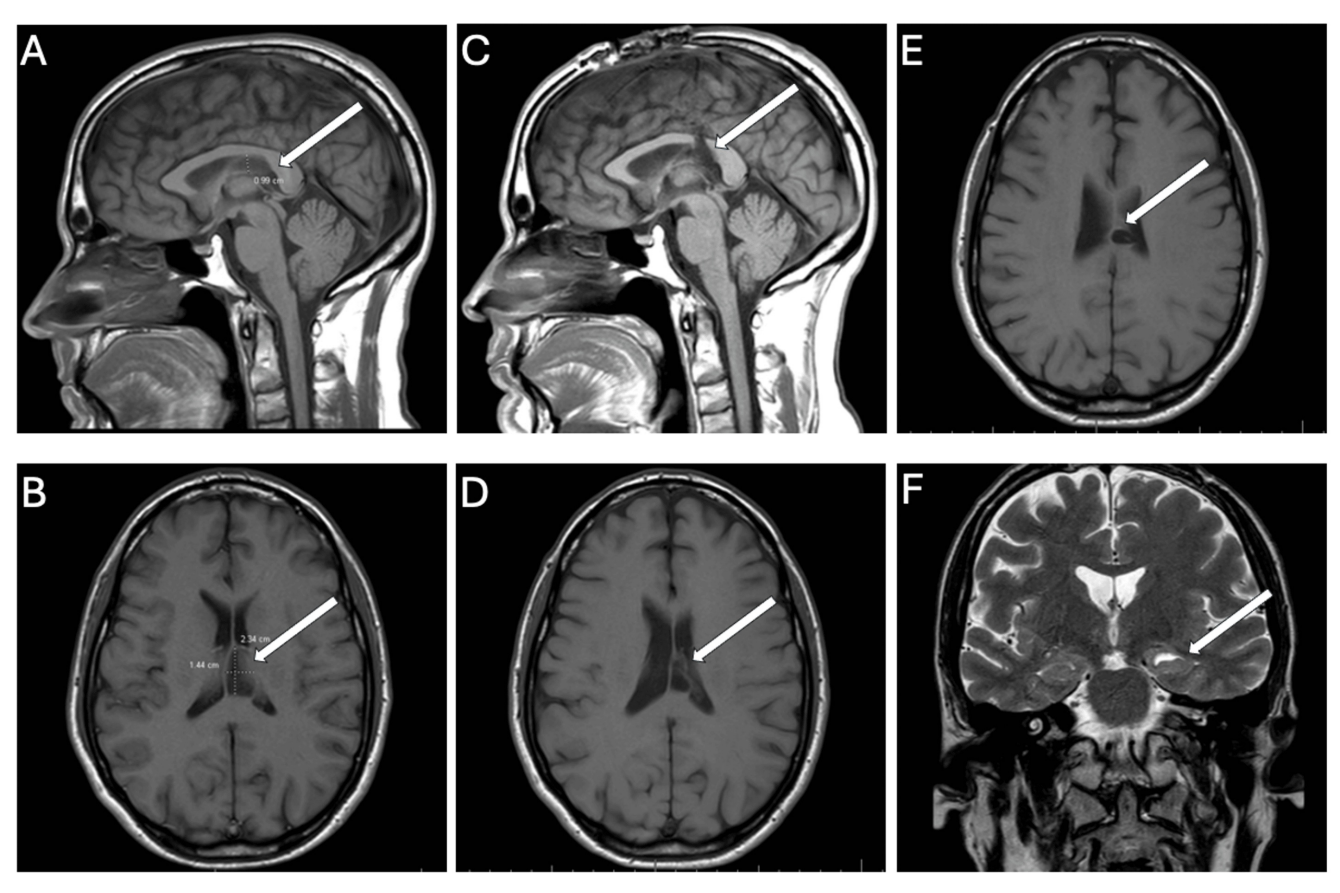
Magnetic resonance imaging (MRI) of the patient’s brain before and after subependymoma resection three years prior to presentation. Panels A and B are T1 sagittal and axial sections demonstrating the location of the initial Grade I subependymoma in the left third ventricle (marked by white arrows). Panels C, D, and E are T1 sagittal and axial sections at the time of hospital presentation demonstrating encephalomalacia from postsurgical changes and external ventricular drain tract years after resection (marked by white arrows). Panel F is a T2 sequence showing volume loss in the left hippocampus consistent with mild left-sided mesial temporal sclerosis (marked by a white arrow).
